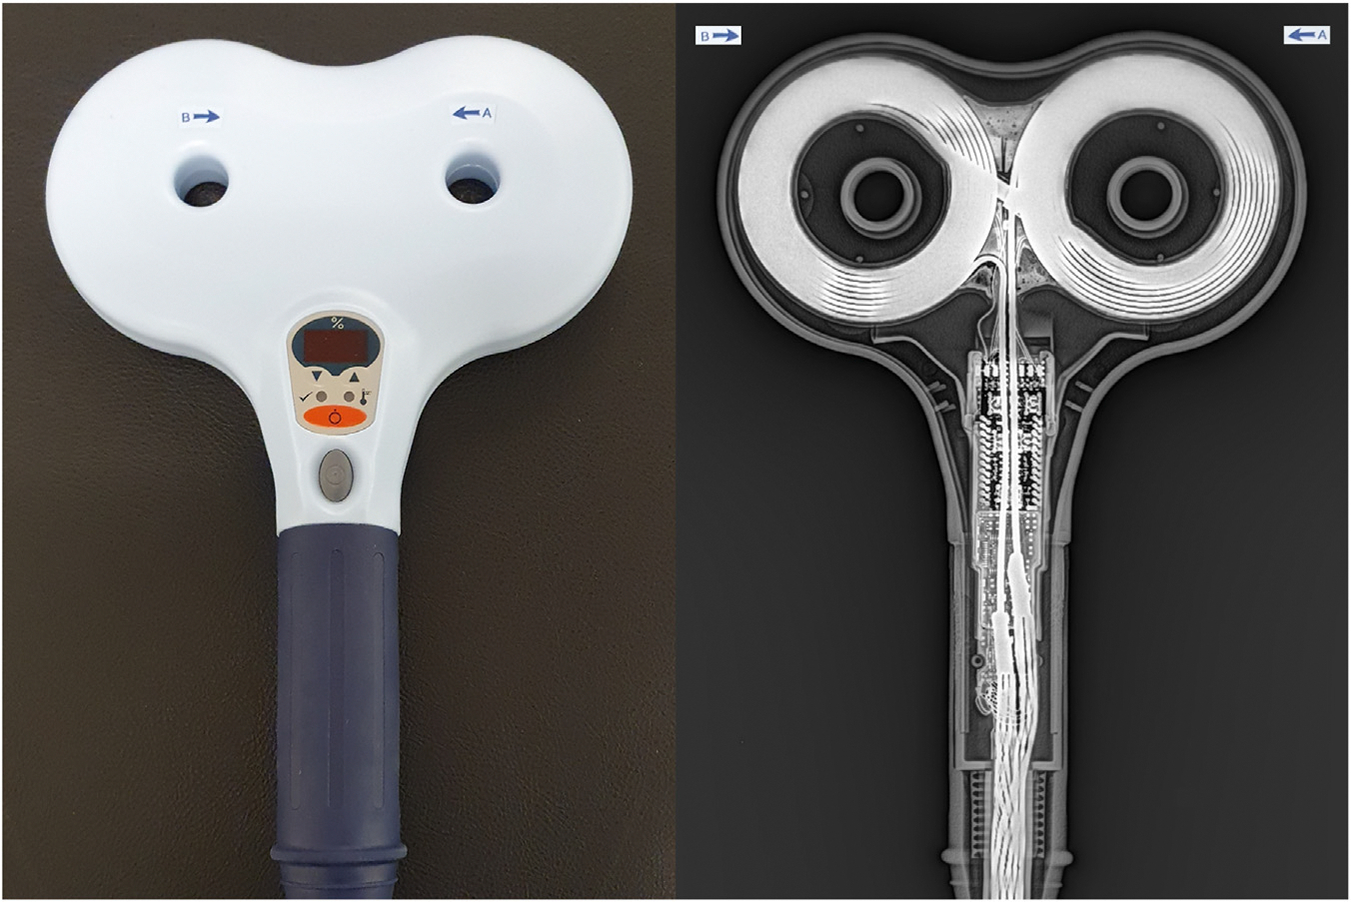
Figure-of-eight transcranial magnetic stimulation coil. (Left panel) Flat figure-of-eight transcranial magnetic stimulation coil. Arrows indicate the initial current direction for this coil (Magstim D70 Remote Coil). Photograph shows operator view of coil, i.e., coil face oriented away from camera. (Right panel) Compound x-ray image of the same transcranial magnetic stimulation coil showing coil windings. Coil face oriented toward x-ray source.
